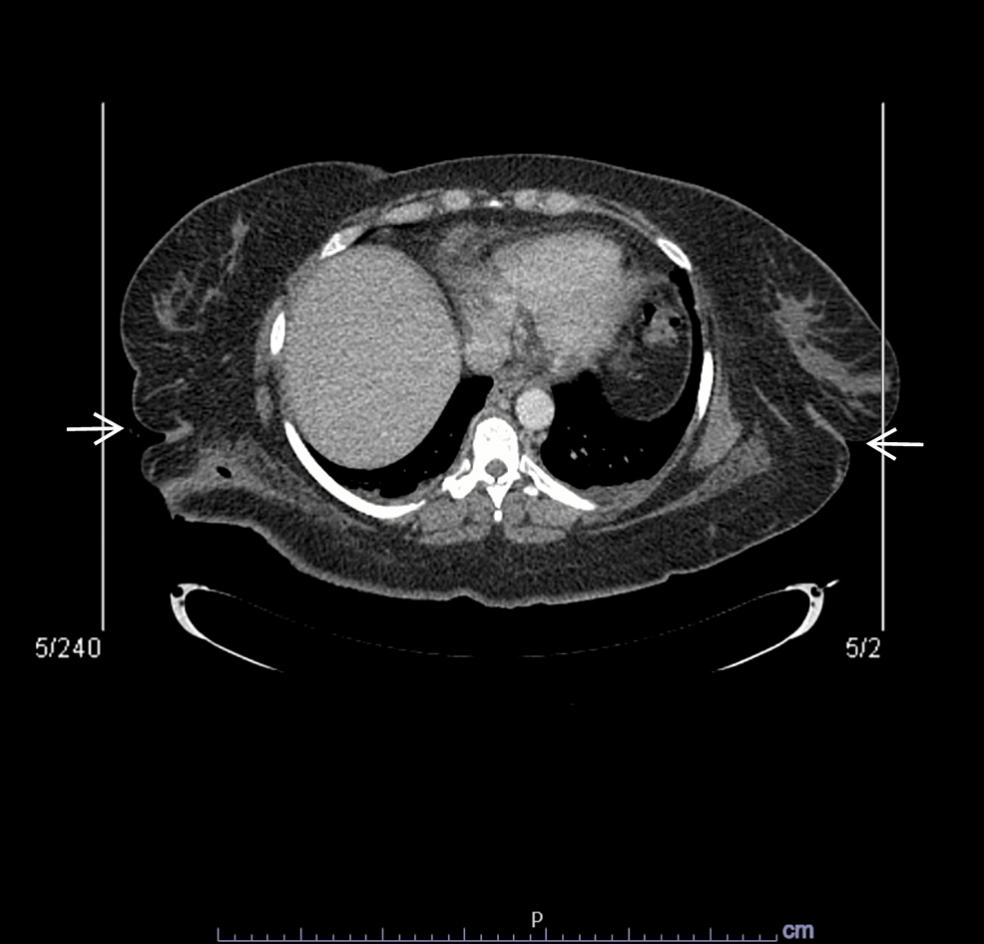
CT scan of the abdomen and pelvis
